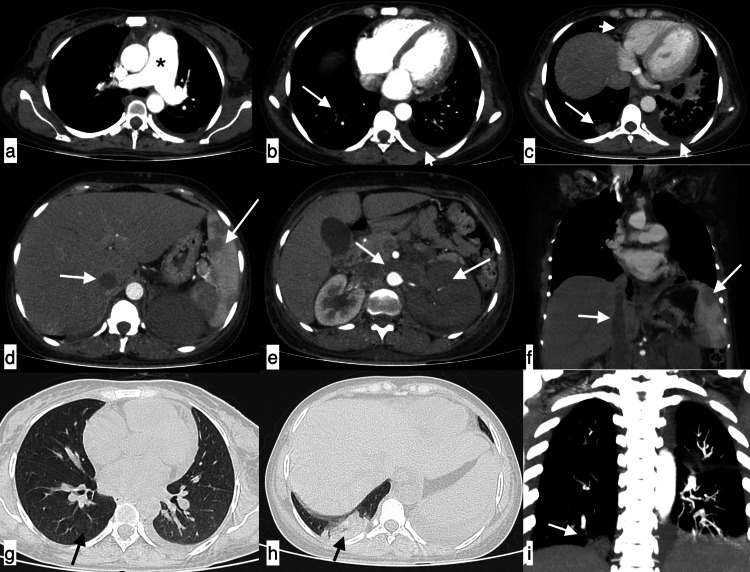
Pulmonary thromboembolism A 28-year-old female patient with SLE presented with complaints of shortness of breath and chest pain of three-day duration. CT pulmonary angiogram revealed a dilated main pulmonary artery (a, asterisk) with hypodense filling defects in the right lower lobar and segmental arteries (b, white arrow), with resultant peripheral subpleural wedge-shaped non-enhancing infarct in the postero-basal segment of the right lower lobe (c, white arrow; g, h, black arrows). Additional findings included thrombosis of the IVC (d, f, white arrows) and left renal vein with a bulky, non-enhancing left kidney (e, white arrows), splenic infarcts (d, f, white arrows), and mild pericardial and left pleural effusion (b, c, arrowheads). Findings were consistent with pulmonary thromboembolism. SLE: systemic lupus erythematosus; HRCT: high-resolution CT; IVC: inferior vena cava
